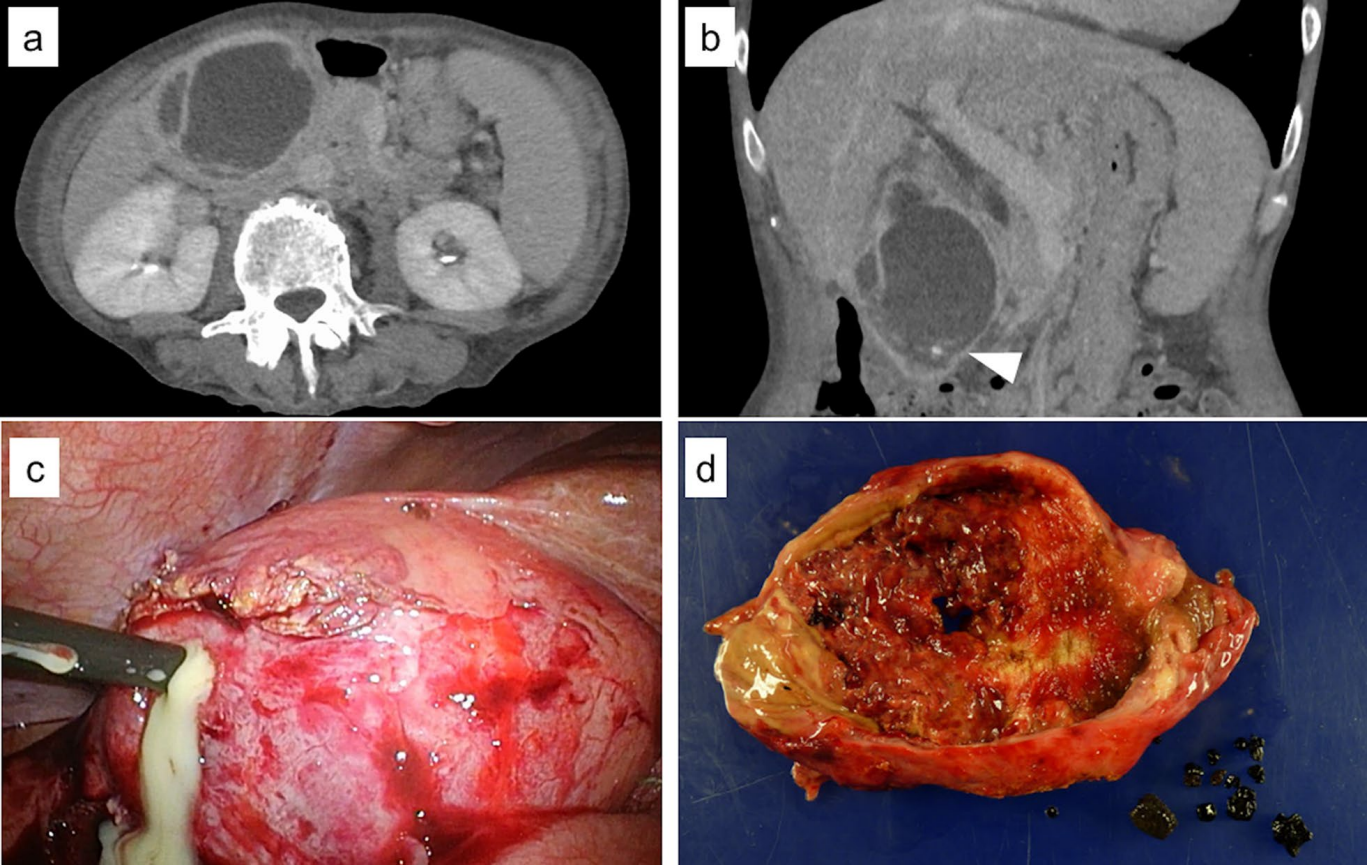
CT scan and gallbladder of initial surgery. Abdominal CT performed at the time of diagnosis of cholecystitis shows marked gallbladder swelling and intramural abscess, with gallstones (arrowhead): a axial and b coronal views. c Laparoscopic cholecystectomy for acute cholecystitis. Iatrogenic perforation of the gallbladder due to the pus drainage. d Gallstones and gallbladder with wall thickening and mucosal necrosis
